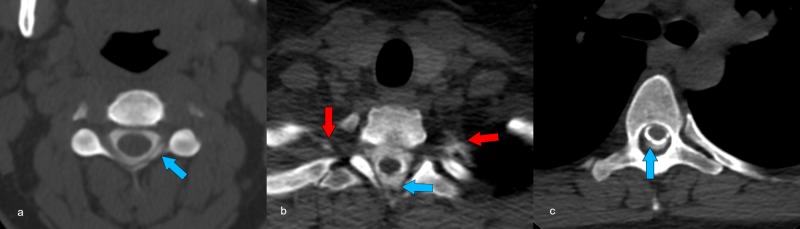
Pre-operative CT myelogram Multiple axial CT images acquired after intrathecal injection of contrast under fluoroscopy demonstrate epidural contrast (blue arrows) spanning from the C3–4 level (a) to the T7 level (c). Contrast flow was so brisk that it is already seen diffusing into the intercostal space (red arrows) at the T2–3 level. Abbreviation: CT, computed tomography.
